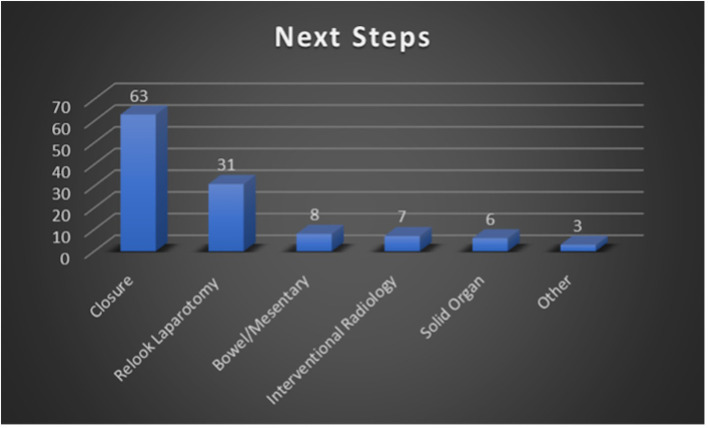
Summary of next step management performed following post-DCL CT. These categories are non-exclusive (e.g., one patient could have undergone both surgical and IR intervention).
